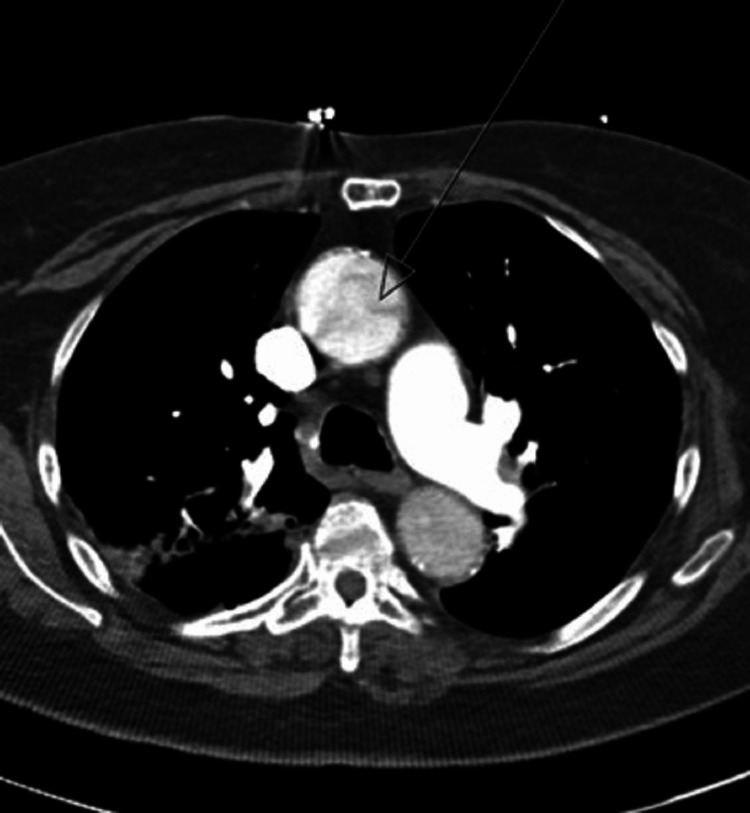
CT angiogram of thoracic aorta concerning for dissection.
